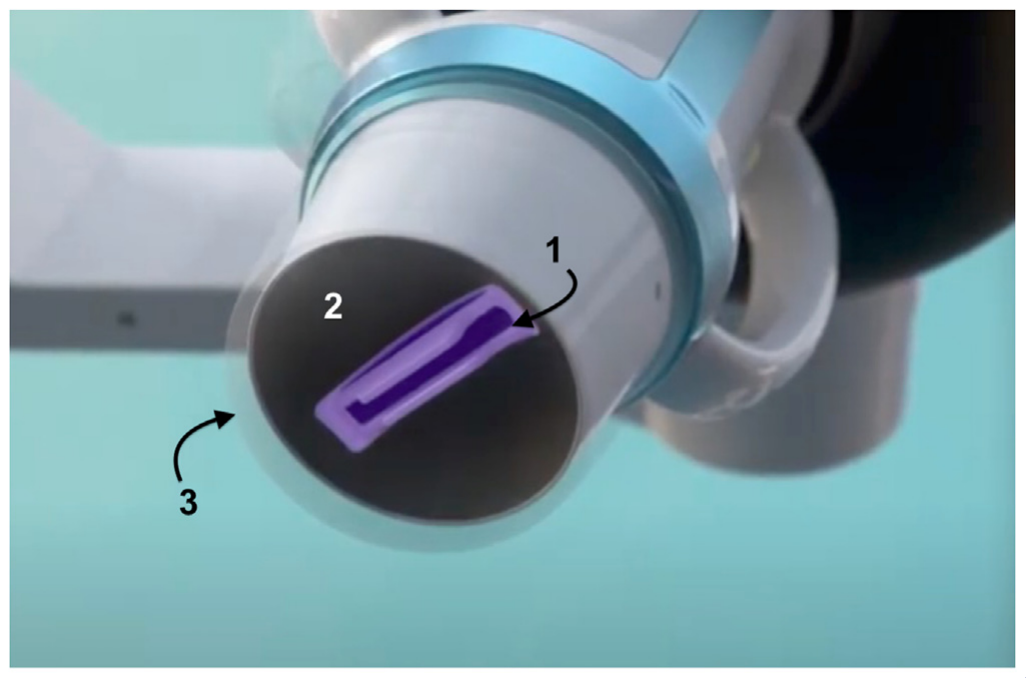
SONOVEIN HD. Visualization and treatment unit (VTU) detail (1) Linear array ultrasound probe (10 MHz). (2) Piezoelectric dome-shaped transducer. (3) Single-use balloon-shaped membrane cooling system (courtesy of Theraclion).
